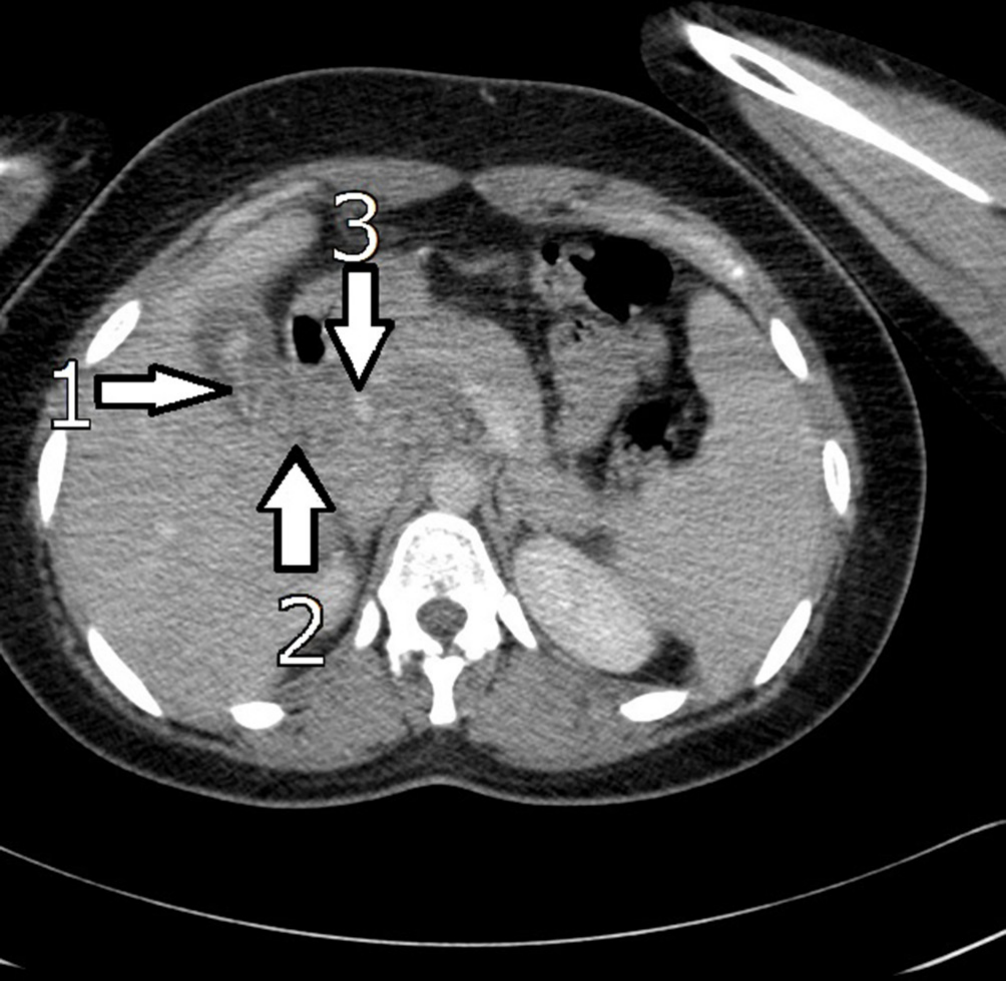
CT abdomen and pelvis with contrast. 1 – high-density signal from worm inside the GB. 2 – worm extending into the proximal CBD. 3 – oedematous pancreatic head. CBD,common bile duct.
